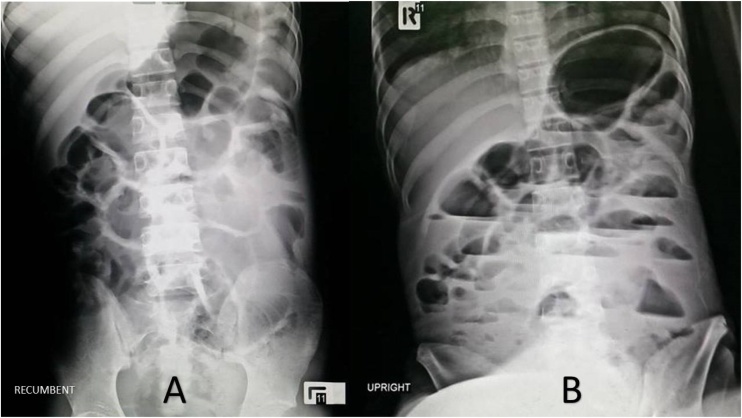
Abdominal radiography revealed dilatation of small and large intestine in recumbent (A) and upright (B) view.
